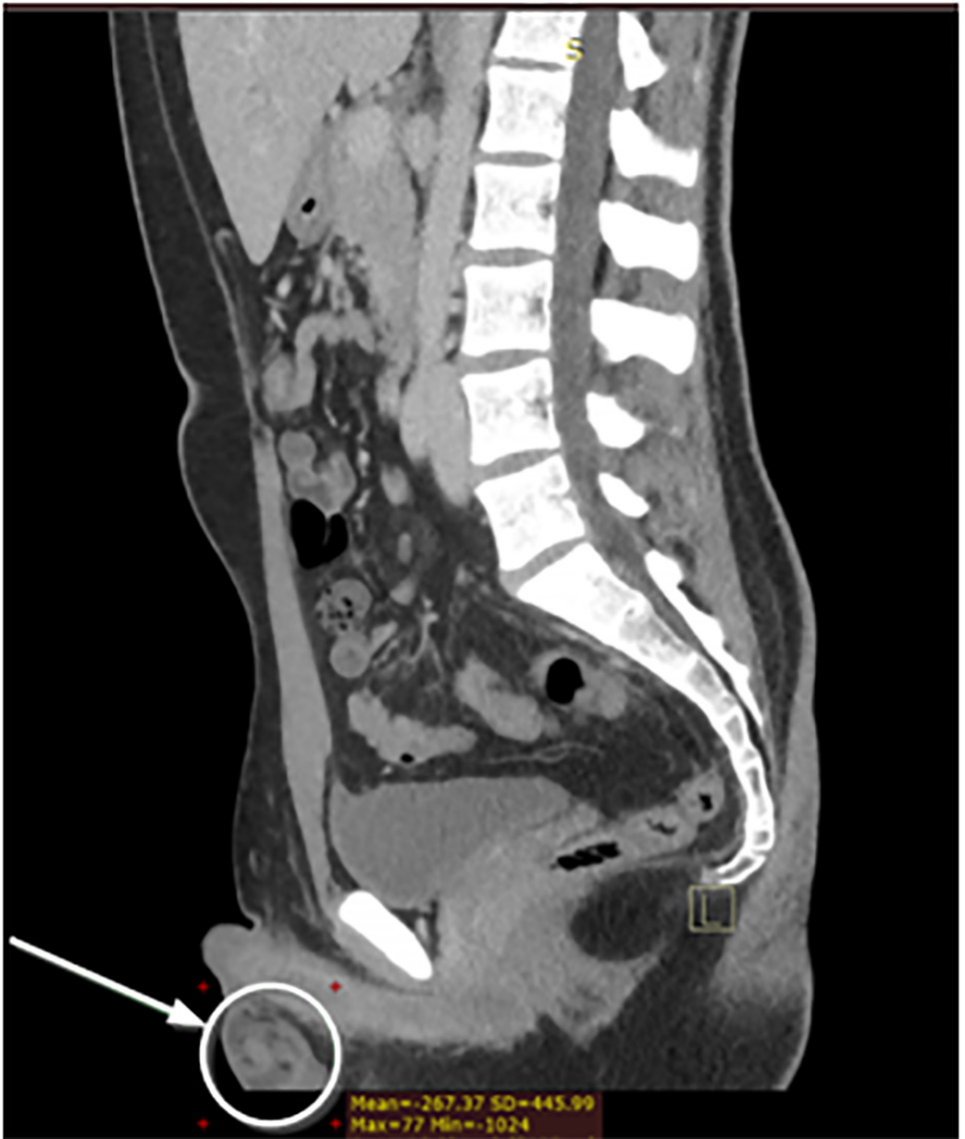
Right parasagittal computed tomography of the abdomen and pelvis showing “whirl” sign consistent with testicular torsion in the right scrotum at the inferior periphery of the image. The white arrow and circle highlight this finding.
